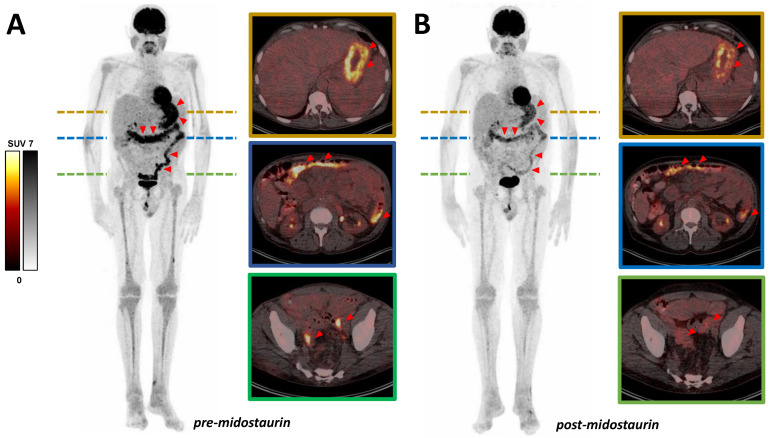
Declining uptake can be seen in presented MIP (maximum intensity projection) and exemplary transversal slices of [^18^F]FDG PET/CT scan in gastrointestinal mastocytosis manifestations. (A) Baseline (blood glucose level: 87 mg/dL; hemoglobin: 11.2 g/dL) and (B) after 6 months’ therapy with midostaurin (blood glucose level: 103 mg/dL; hemoglobin: 11.5 g/dL). Exemplary SUV_peak_ values (pre- vs. post-midostaurin treatment): in stomach 7.2 vs. 5.8 and in colon 6.4 vs. 3.4. Diffuse uptake in bone marrow was also slightly regressive (exemplary in the left tibia SUV_peak_ 2.3 vs. 1.9), while splenomegaly remained similar. Red triangles exemplarily point to gastrointestinal mastocytosis manifestations.
